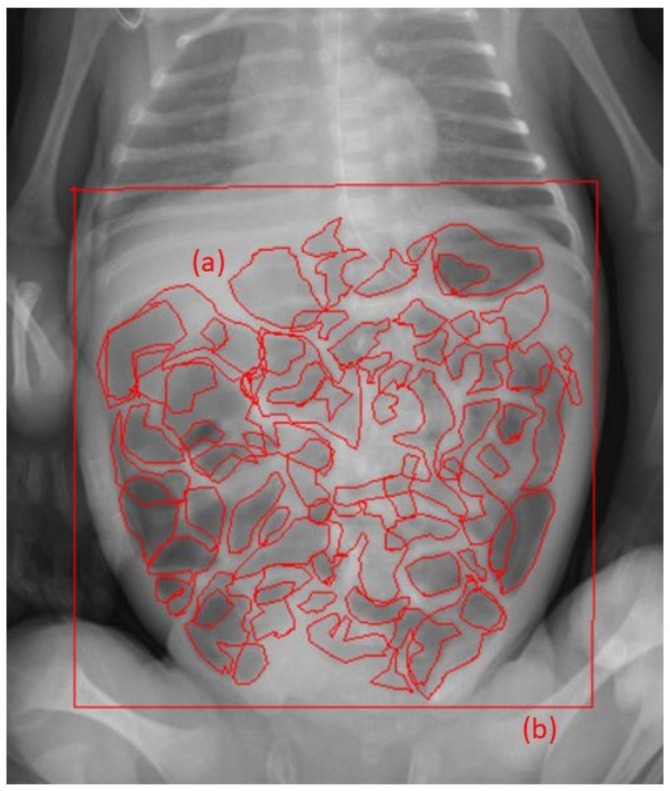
Estimation of gas volume score (GVS) in a plain abdominal radiograph. The outlines of the intestinal gas were manually traced, and the area was calculated by counting the pixel value using ImgWorks; all areas were summed in (a). The pixel count of the rectangular area (window of the abdominal area) is calculated in (b). GVS calculated as (a)/(b) of this image is “46,176.5/97,968 = 0.471”.
